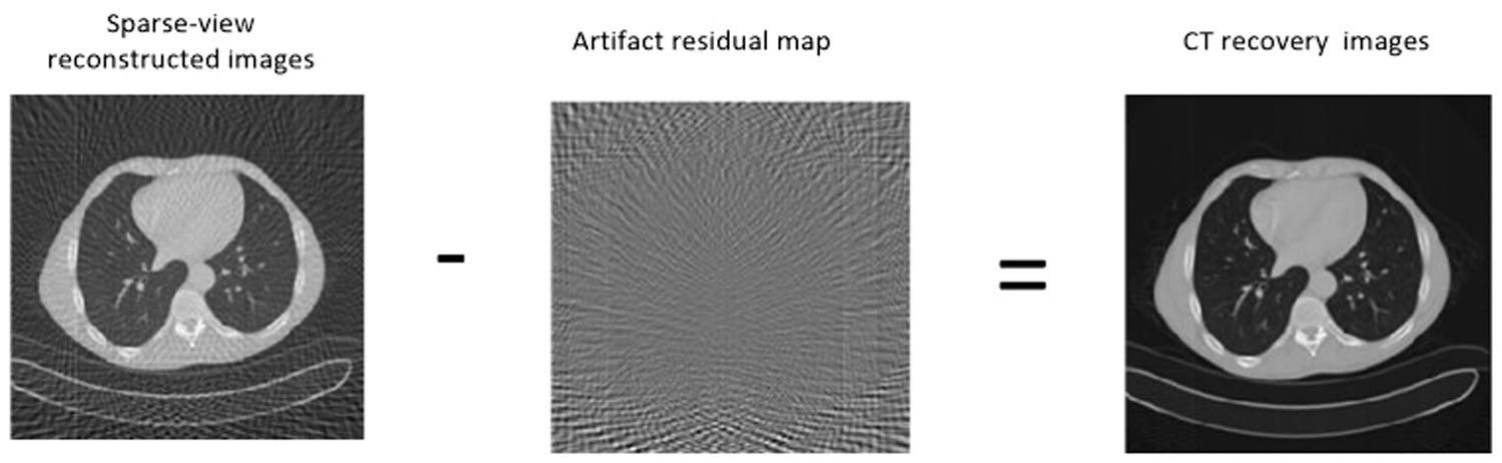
The process of removing artifacts from sparse reconstructed images in CT.
